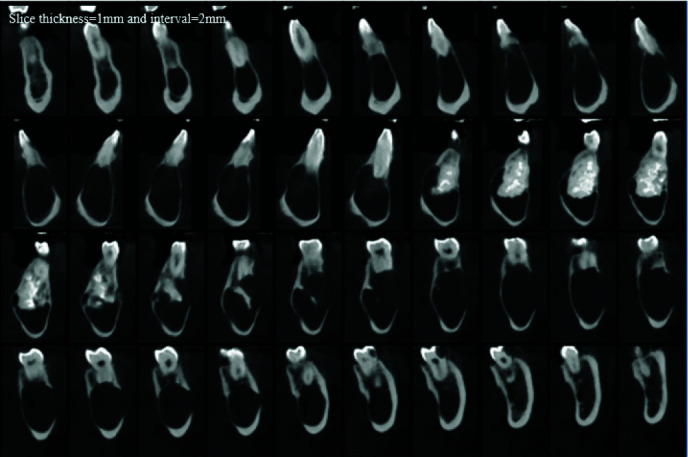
Cone beam computed tomography scan shows thinning of both buccal and lingual cortices and loss of continuity of buccal cortex
